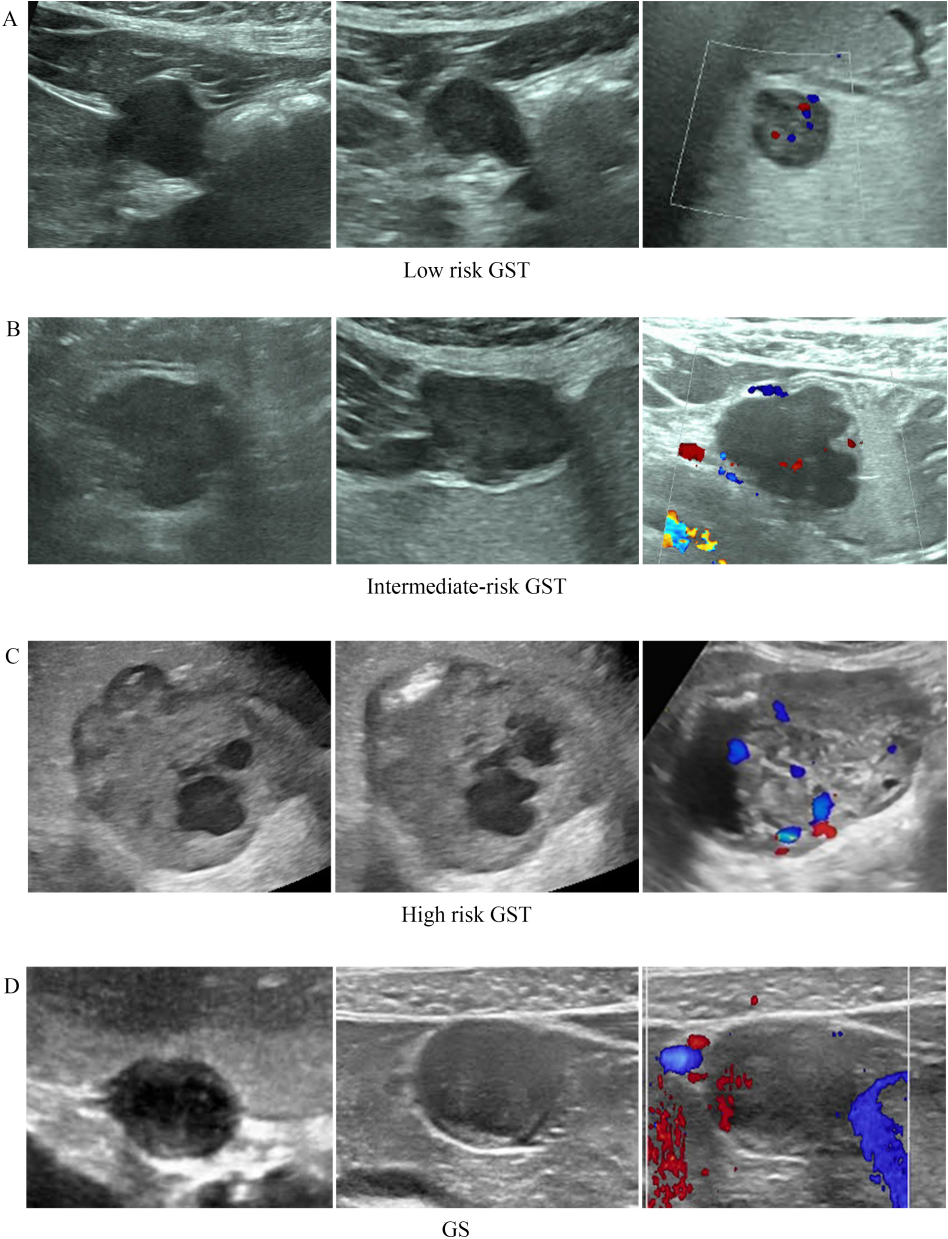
Sonography of different group of GST and GS. (A) The diameter of GST was 2.9cm with regular shape, clear boundary and homogeneous internal echo, tumor was extended into the muscularis propria. The CDFI was grade II; (B) The diameter of GST was 6.2cm with lobular shape, blurred boundary and heterogeneous internal echo, tumor was extended into the muscularis propria. The CDFI was grade II; (C) The diameter of GST was 12cm with irregular shape, clear boundary and heterogeneous internal echo (cystic-solid). The CDFI was grade III; (D) The diameter of GS was 2.5cm with regular shape, clear boundary and homogeneous internal echo. The CDFI was grade I.
